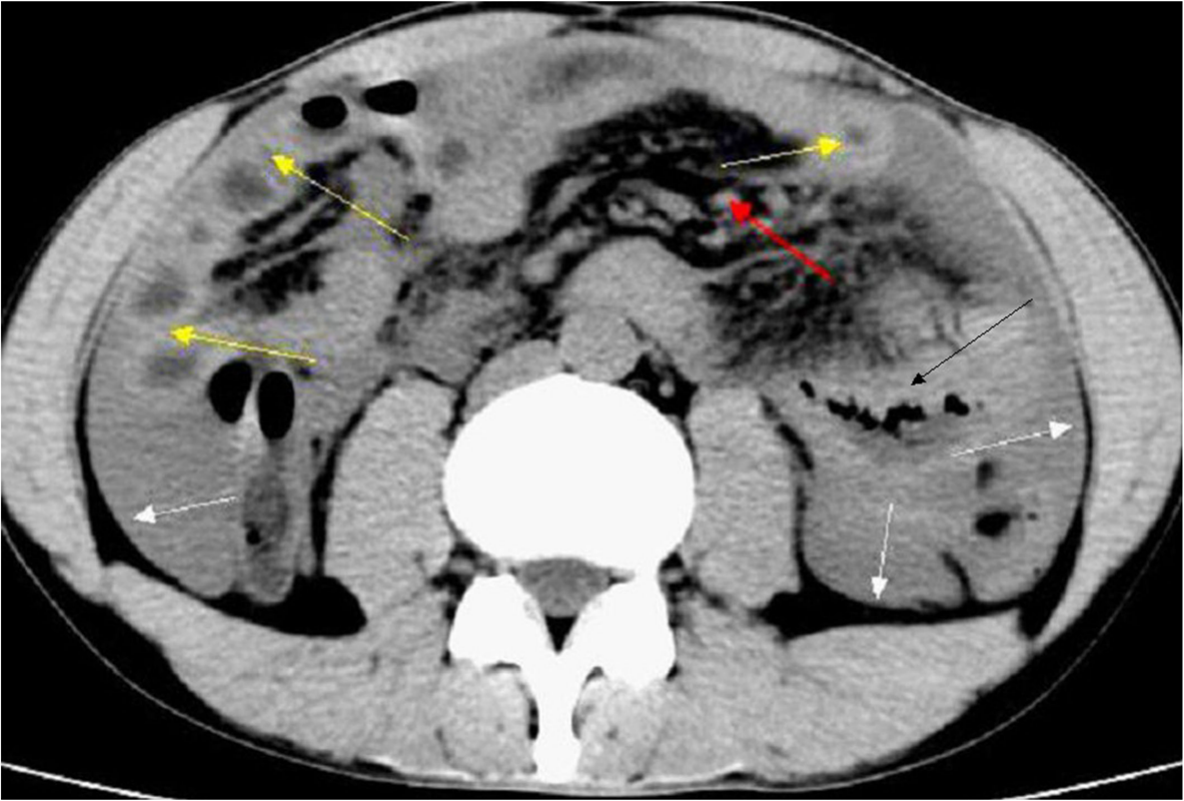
Computed tomography scans of the whole abdomen showed a significantly thickened intestinal wall (yellow arrows) located at the end of the jejunum and the proximal ileum, excessive ascites (white arrows), a few enlarged lymph nodes in the abdomen (red arrow) and the location of the primary angiosarcoma of the small intestine (black arrow).
